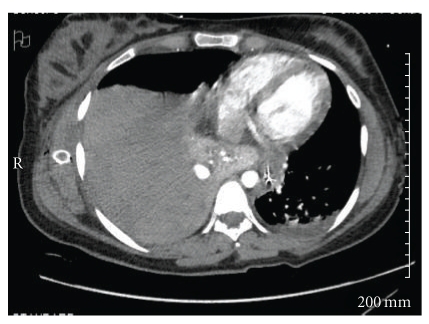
Computed tomography of the chest with I.V. contrast showing right-sided pleural effusion.
